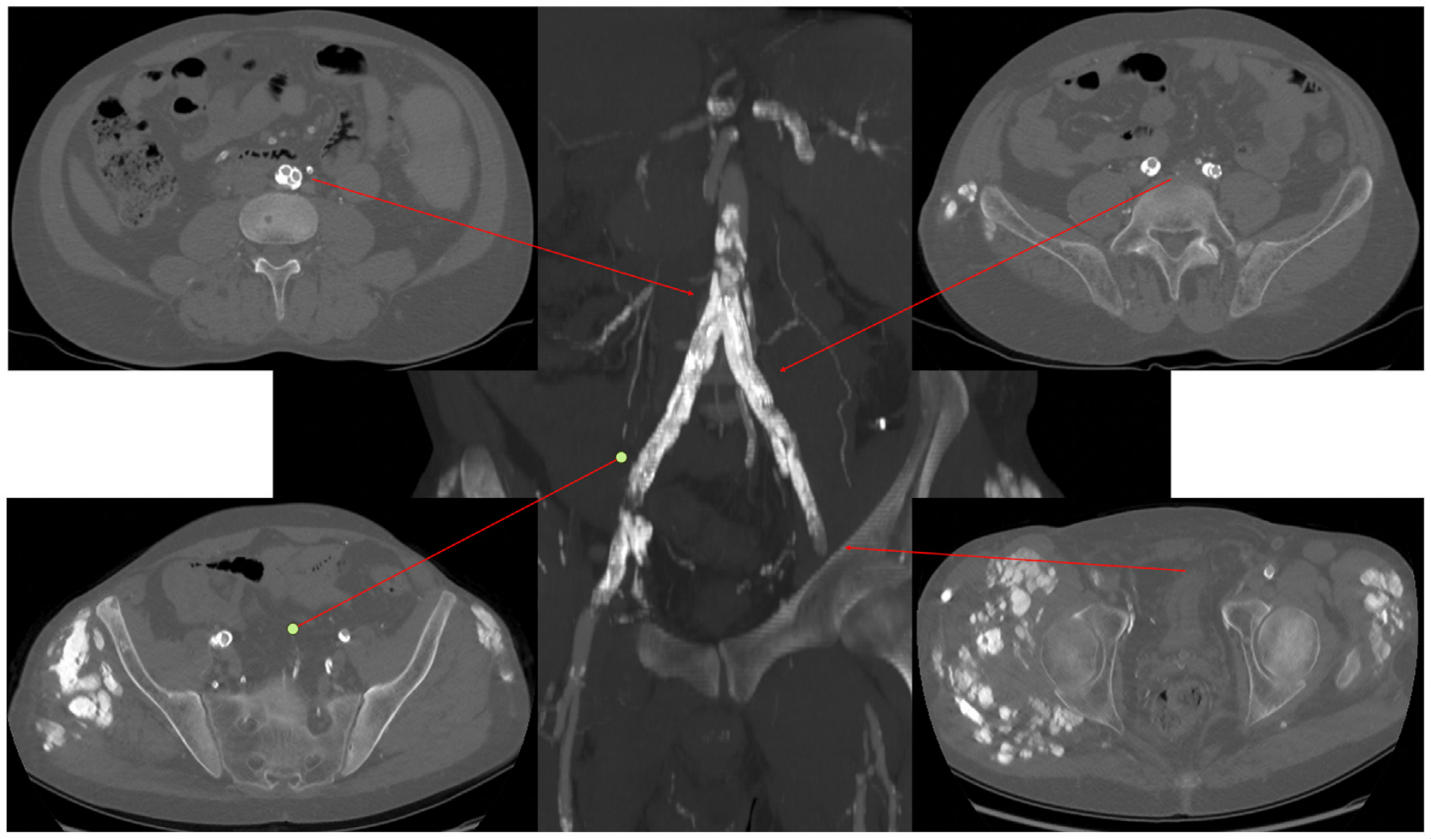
Preoperative computed tomography (CT) scan.
